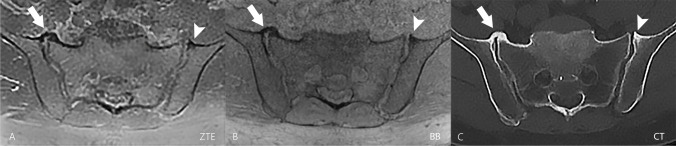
This 72-year-old female patient was referred for tumor staging. The transverse ZTE image (A) showed right-sided bridging osteophytes of the anterosuperior sacroiliac joint (arrowhead) and bone spurs on the left side (arrow) that were also perfectly visible on the BB sequence (B) and the CT scan (C). In this patient, the signal-to-noise ratio was lower in the ZTE and BB sequence since the patient had a high body mass index of 29.4. In comparison, for the CT scan, the required optimal dose to ensure sufficient image quality was tailored to the individual patient by the modern CT scanner. However, the image quality of the ZTE and BB sequence is still sufficient to characterize the SI joint bone findings. Abbreviations: ZTE = zero echo time; BB = black bone; CT = computed tomography; SI = sacroiliac joint
